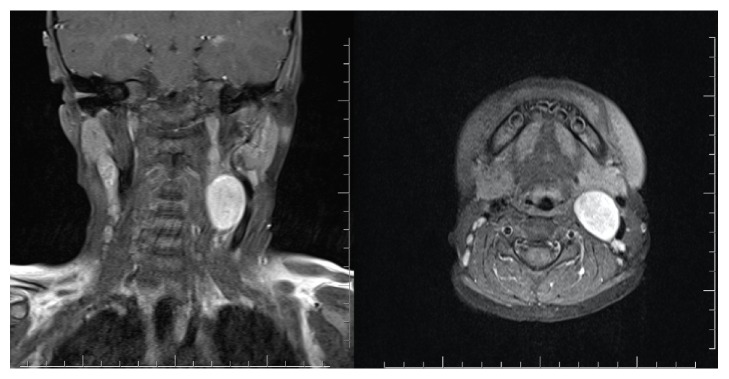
MRI of the head and neck: axial and coronal views of mass. The lesion with 2,8*∗*2,3 cm size on the front of musculus sternocleidomastoideus and internal carotid artery.
